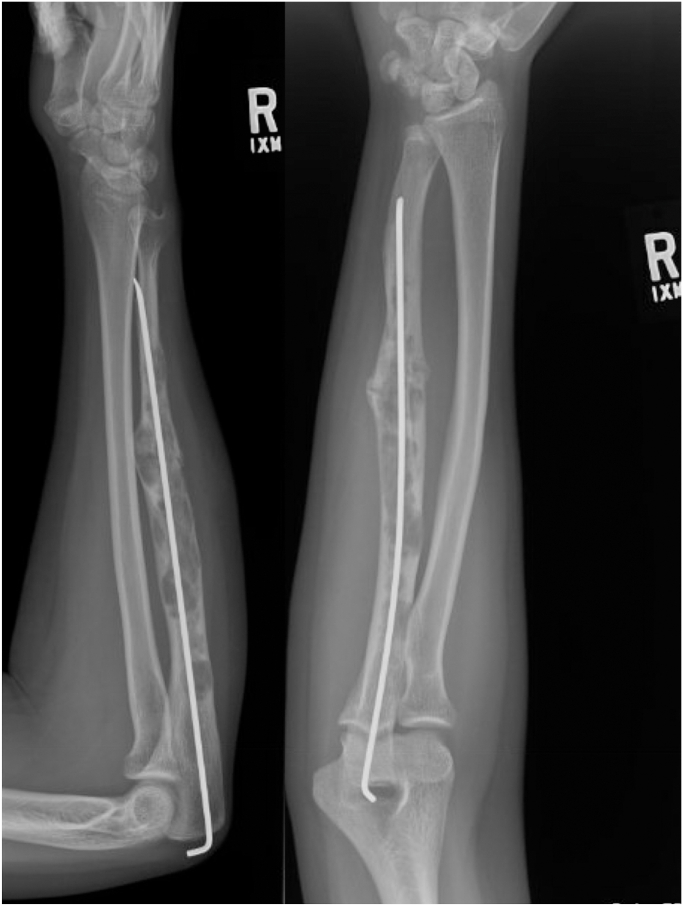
Patient at age 15. One-month postoperative radiograph status post right ulna open biopsy and intramedullary nailing for pathologic fracture.
